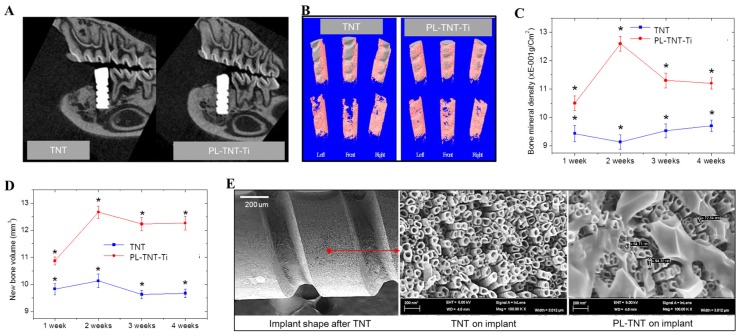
The microcomputed tomographic (µ-CT) analyses of peri-implant tissue at 1, 2, 3 and 4 weeks after implantation at the healed edentulous mandibular site. (A) Two-dimensional µ-CT analysis of peri-implant tissue at 4 weeks after implantation; (B) Three-dimensional µ-CT analysis of peri-implant tissue at 4 weeks after implantation (the gray color represents the implant whereas the pink and yellow colors represent the bone tissue); (C) Three-dimensional µ-CT analysis of bone mineral density around the implant surface; (D) Three-dimensional µ-CT analysis of the volume of the new bone formation around the implant surface (* *p* < 0.05); and (E) surface morphologies of implant.
